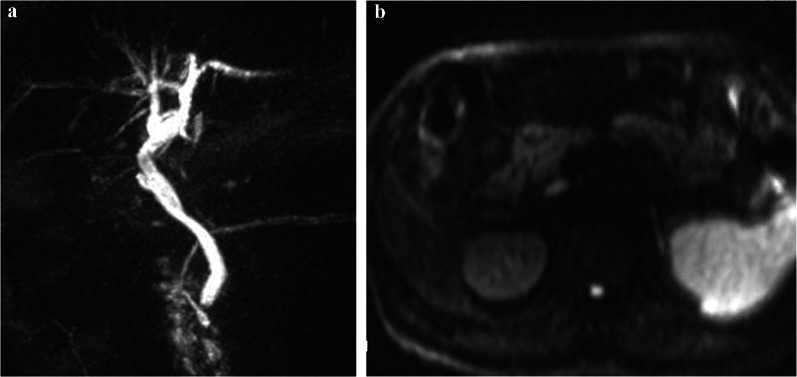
a Postoperative MRI image of the biliary tree. b No evidence of malignancy in the diffusion mode of postoperative MRI
